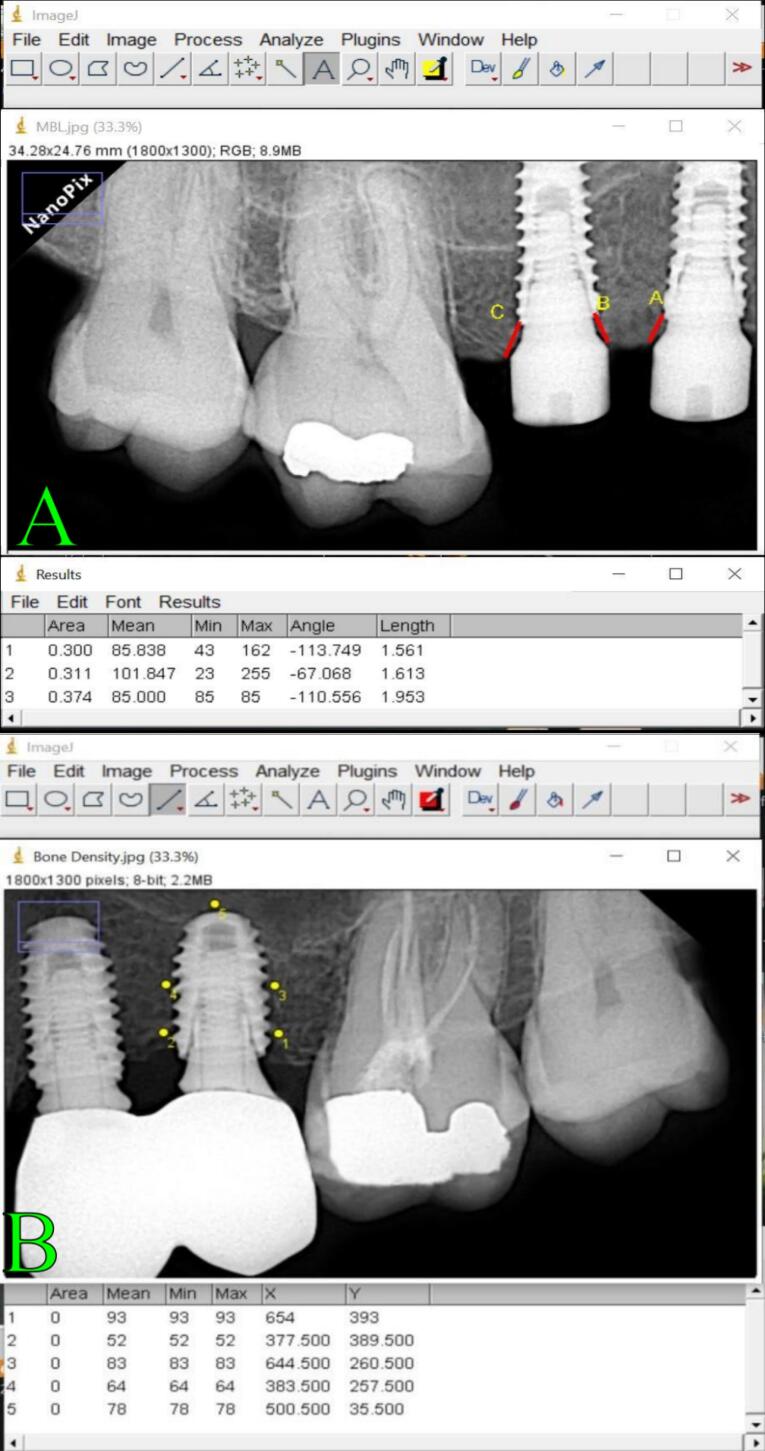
The marginal bone loss (MBL) was measured using periapical radiographs, which were taken at the beginning and 1, 3, and 6 months following implant insertion. Radiographs were obtained using a digital sensor and the PRO 70X Intra radiography device, which operates at 70 kVp and 8 mA (Figure 3B).
